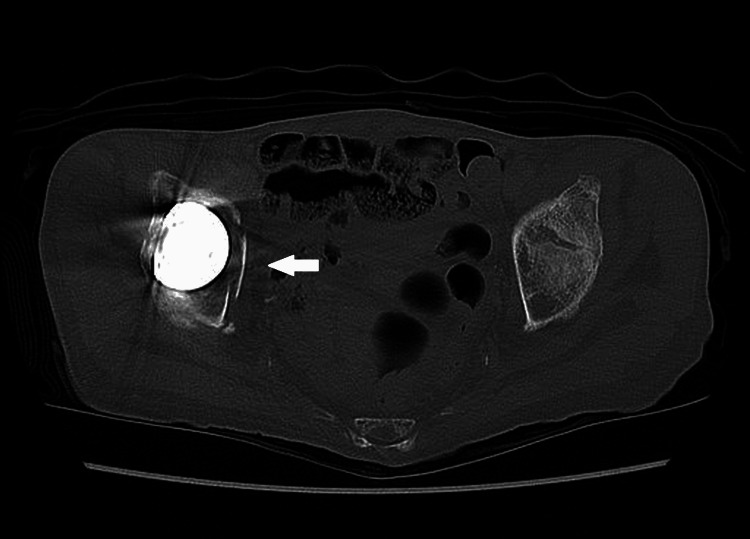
Pelvic CT axial image on admission. A transverse acetabular fracture was suspected based on findings from CT scans (arrow).
